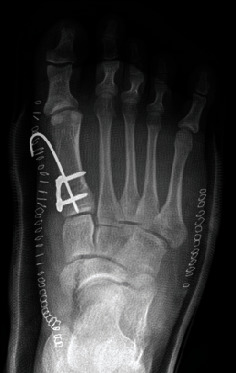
Postoperative posteroanterior radiograph, including plate and K-wire osteosynthesis.
